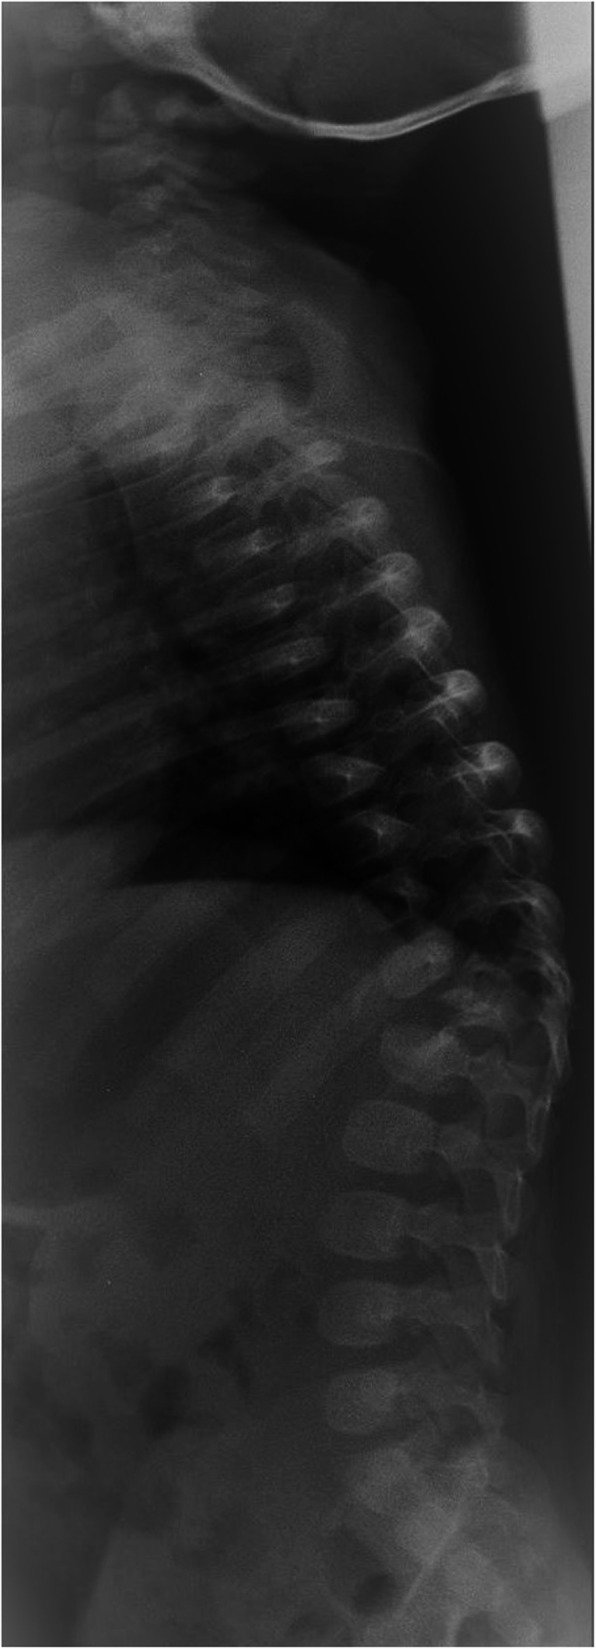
Lateral radiograph at 6 months of age shows the almost complete destruction of T12 vertebral body, resulting in angular kyphosis above 50°
